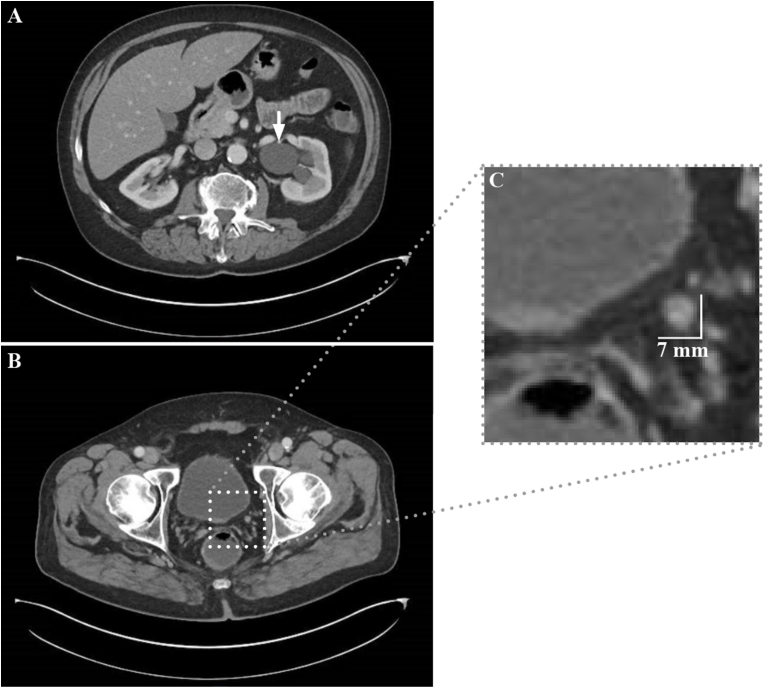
Computed tomography (CT) of the abdomen and pelvis. Cross-sectional imaging using computed tomography (CT) of the abdomen and pelvis with IV contrast shows marked left hydroureteronephrosis and an enhancing ureteral mass in the distal left ureter.
